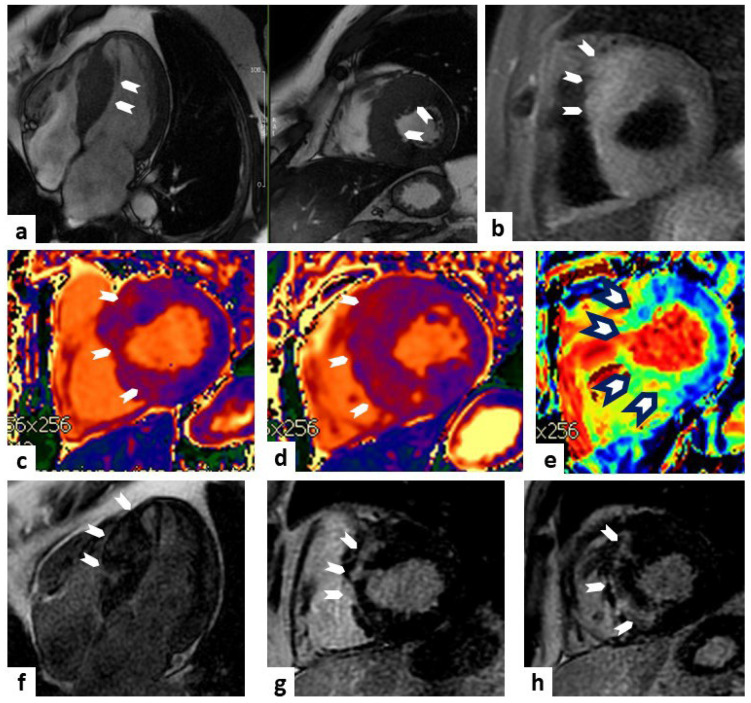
Cardiac MRI of hypertrophic cardiomyopathy (HCM). A case of a 38-year-old male with a history of premature ventricular beats. Panel (a) (SSFP sequences) represents a four-chamber and short axis views (top left panel and top middle panel, respectively) showing a marked and asymmetric hypertrophy of the interventricular septum (white arrowheads). Panel (b) (TIR t2 sequence) represents a short-axis view showing a higher signal al the level of the septum (white arrowheads) compared to the remote myocardium. Panels (c,d) represent increased values on T1 mapping sequences at the level of the basal and middle septum (white arrowheads), respectively. Panel (e) represents increased values on extracellular volume (ECV) sequence at the level of middle septum (white arrowheads black outlines). Panels (f–h) (LGE sequences) display a non-ischemic pattern of hyperenhancement at the level of septum (white arrowheads). Taken together, these findings are diagnostic of asymmetric HCM.
